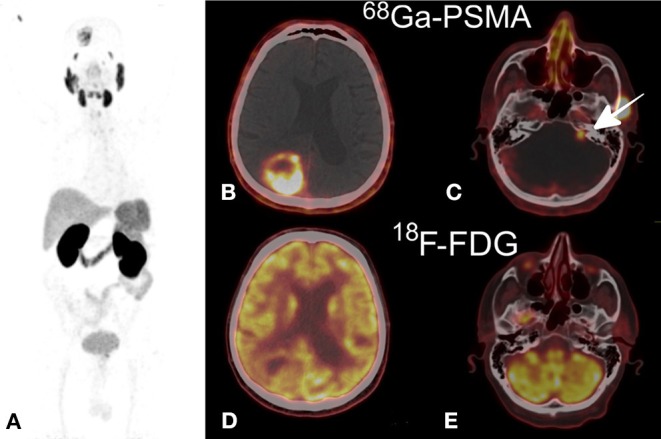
(A) Maximum intensity projection ^68^Ga-PSMA (MIP), (B,C)
